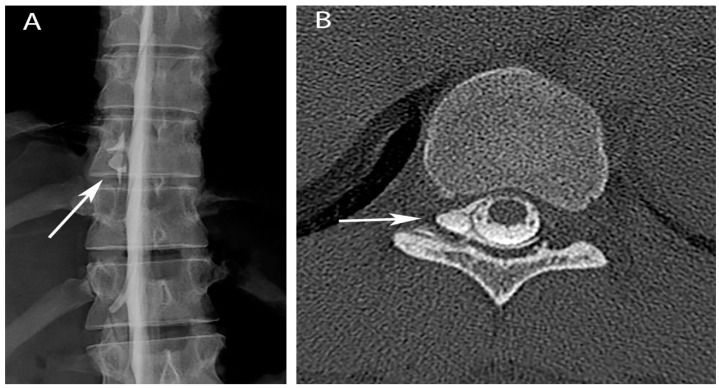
Myelogram (A) and post-myelogram CT (B) show extravasation of contrast (arrows) at the T10-11 neural foramen (right sided extravasation due to patient positioning).
